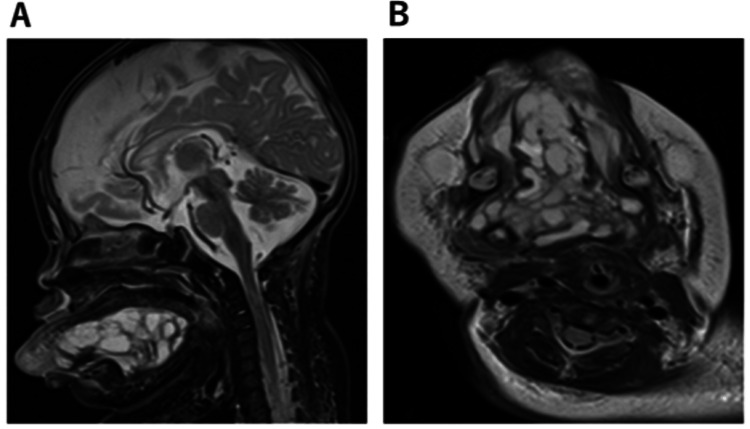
MRI (A) Sagittal plane on magnetic resonance imaging (MRI). (B) Horizontal plane on MRI. The figure shows a multilocular cystic mass, measuring approximately 6 × 5 × 3 cm in diameter, on the floor of the oral cavity and under the tongue.
